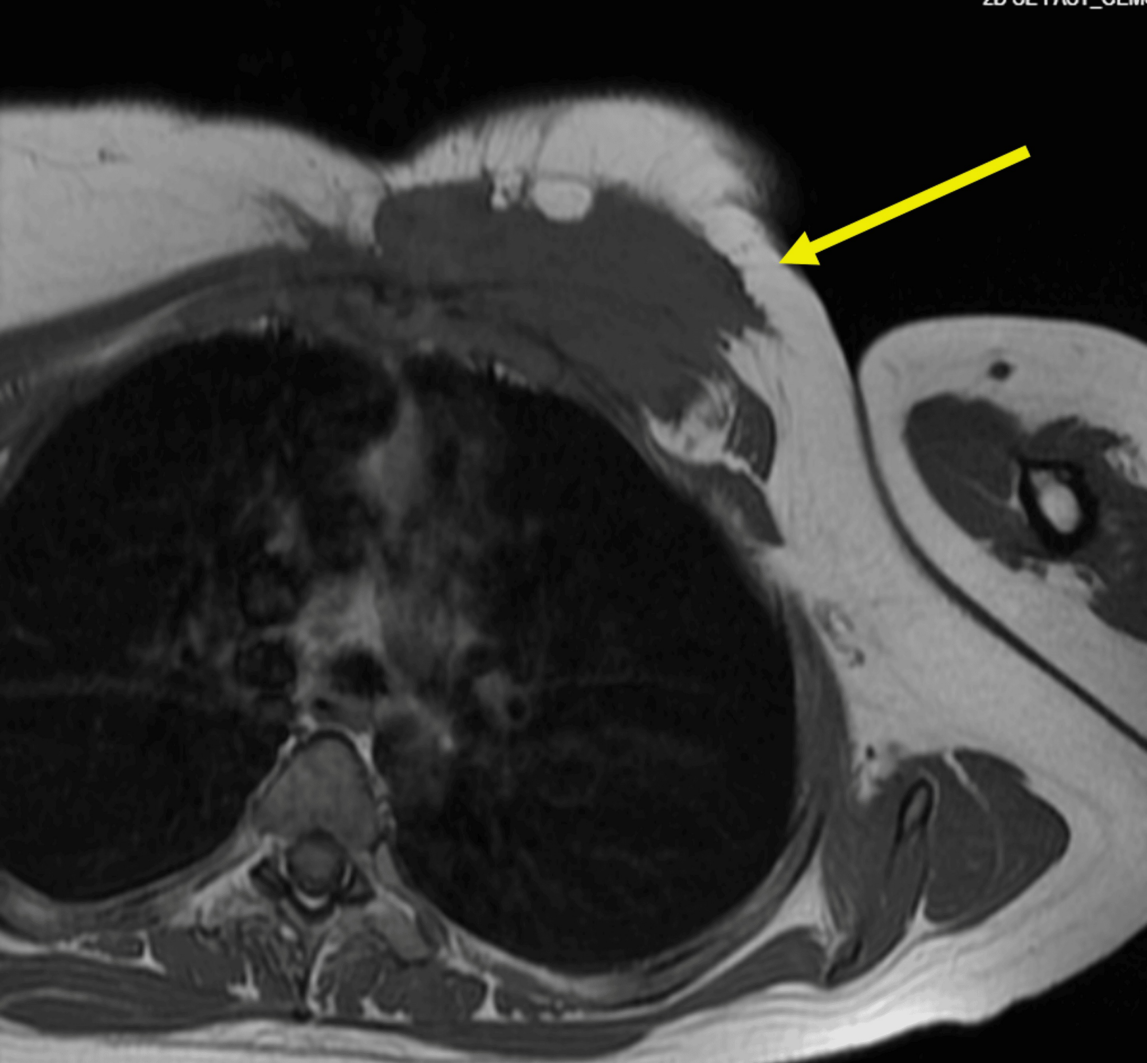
MRI of left chest wall mass involving the left pectoralis major muscle, underlying costal cartilage junctions, and abuts the anterior pleura.
